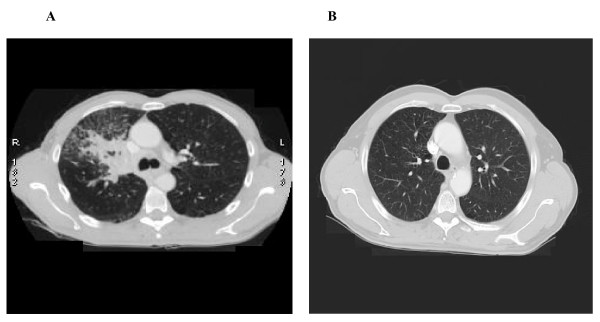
(A) CT scan June 2008 before therapy. (B) CT scan April 2009 after therapy.
